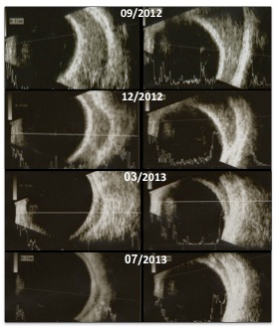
Consecutive ultrasounds of the left eye – superior transversal view (left column) and lateral transversal view (right column) – documenting progressive resolution of the lacrimal gland lesion after radiation therapy treatment was performed in November 2012.
